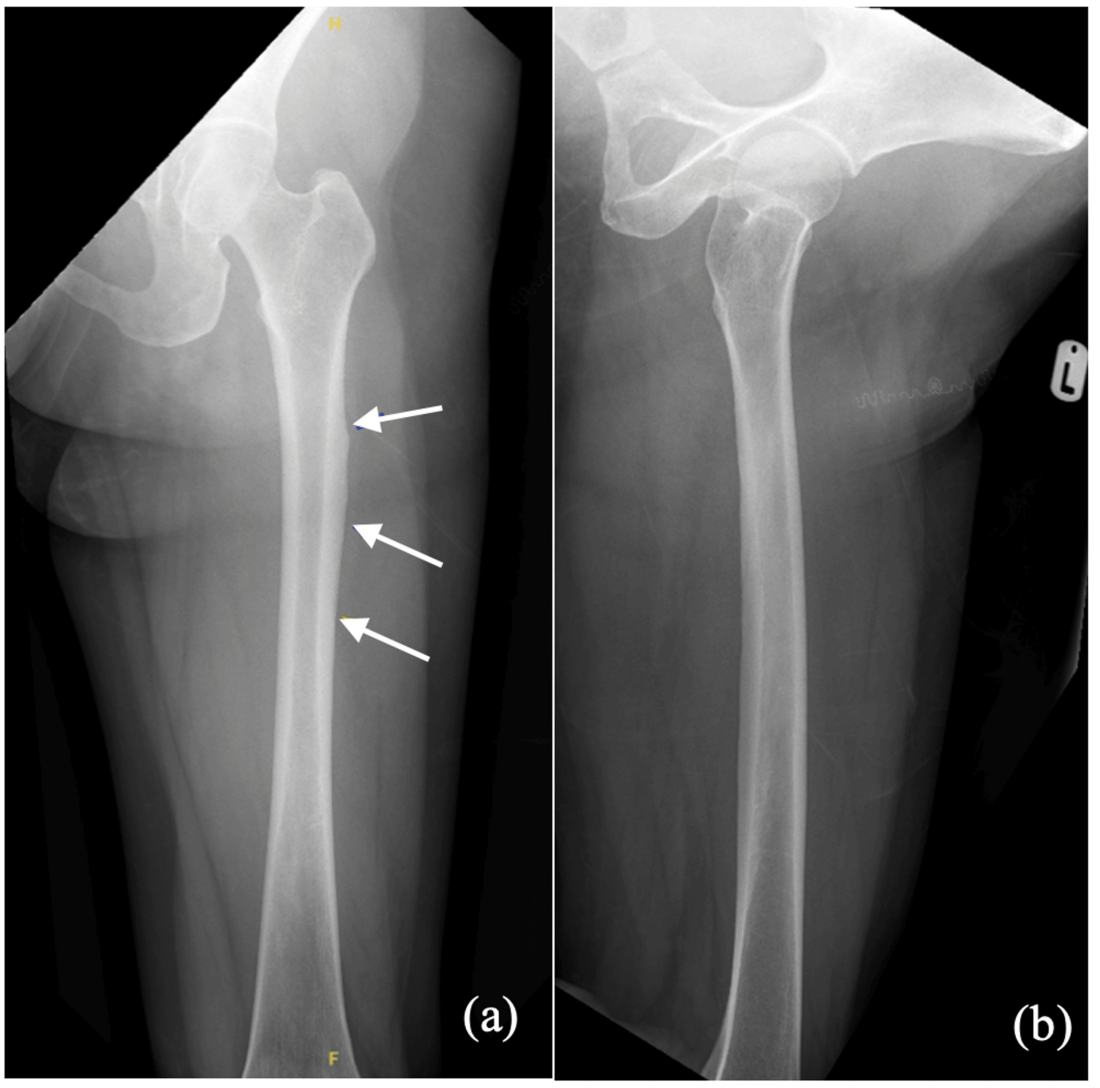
Radiographs of the left femur showing multiple periosteal reactions along the shaft (solid arrows). (a) AP view and (b) lateral view
